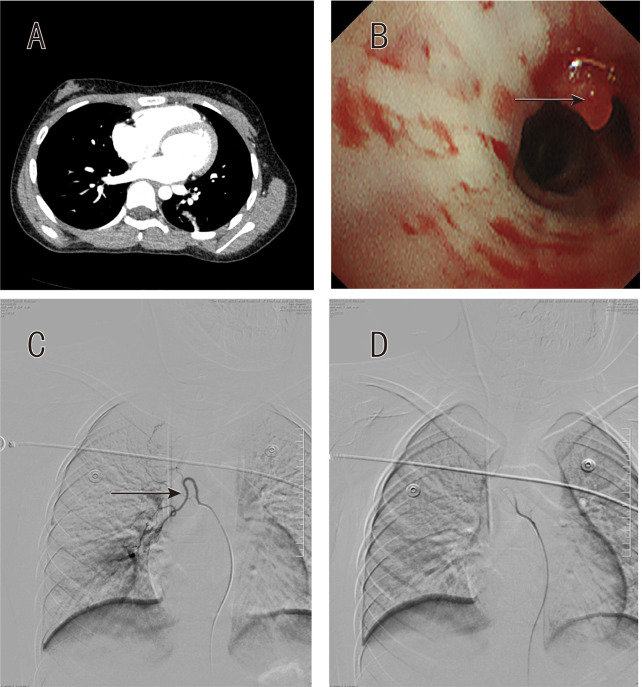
Patient 1 diagnostic and therapeutic sequence. (A) Computed tomography angiography showing no definitive vascular abnormality. (B) Bronchoscopic image demonstrating localized mucosal elevation in right lower lobe lateral basal segment without pulsation (arrow). (C) Selective right bronchial arteriography revealing tortuous vessels with chaotic branching pattern. (D) Post-embolization angiography confirming successful occlusion with absent distal flow.
